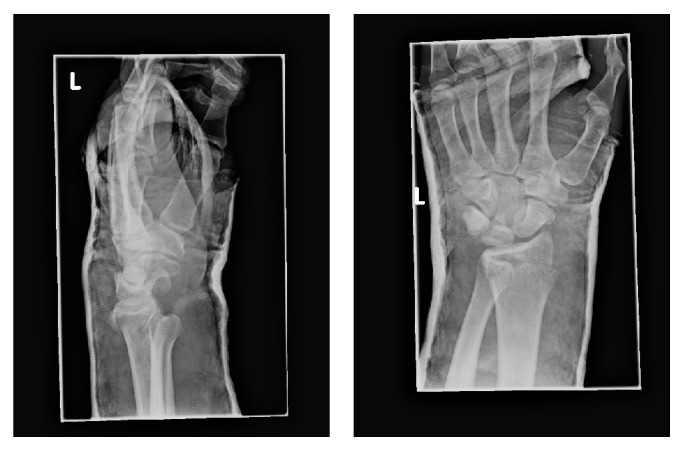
Anteroposterior and lateral X-rays of the affected wrist postimmobilization.
